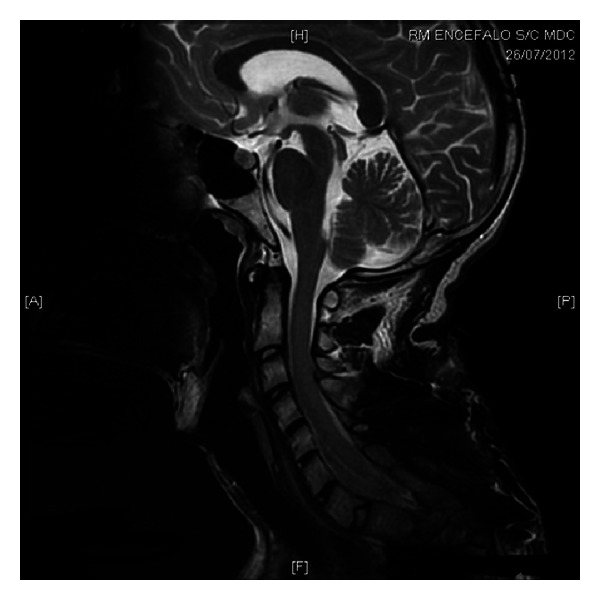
Cervical magnetic resonance imaging showing the presence of a fistulous tract and a fluid pocket in the subcutaneous tissues overlying C4.
